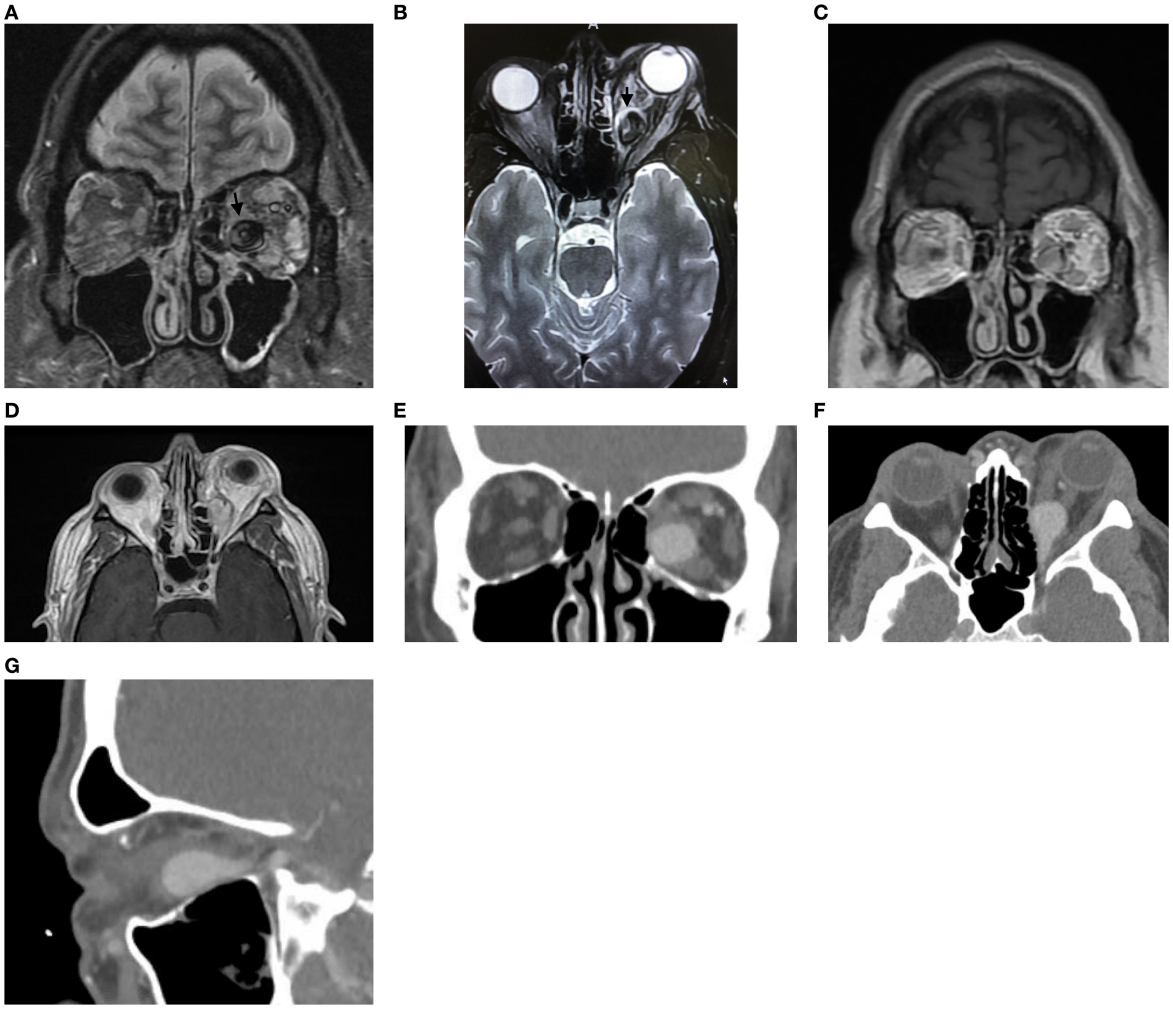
(A) Coronal transverse relaxation time short tau inversion recovery magnetic resonance image demonstrating a large venous aneurysm in the inferomedial left orbit. (B) Axial transverse relaxation time short tau inversion recovery magnetic resonance image demonstrating a dilated venous aneurysm in the posterior medial left orbit (arrow) with associated edema of the medial and lateral rectus muscles. (C, D) Longitudinal relaxation time post-gadolinium magnetic resonance coronal (C) and axial (D) images demonstrating enhancement of the venous aneurysm in the inferomedial left orbit. (E–G) Computed tomography angiography with intravenous contrast in coronal (E), axial (F), and sagittal (G) views demonstrating enhancement of the venous aneurysm, which extends to the orbital apex.
